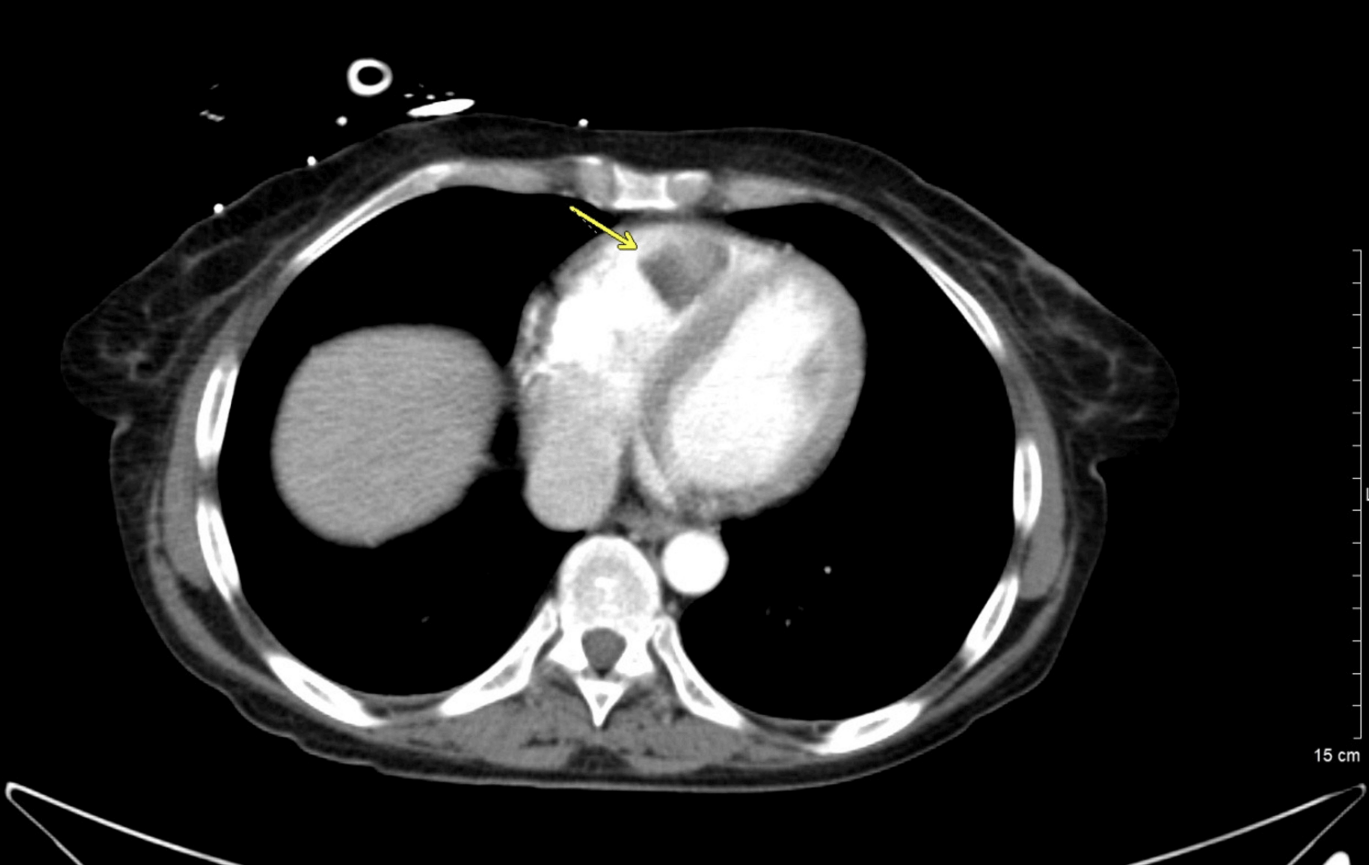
Initial axial post-contrast CT of the chest showing right ventricular filling defect (arrow).
